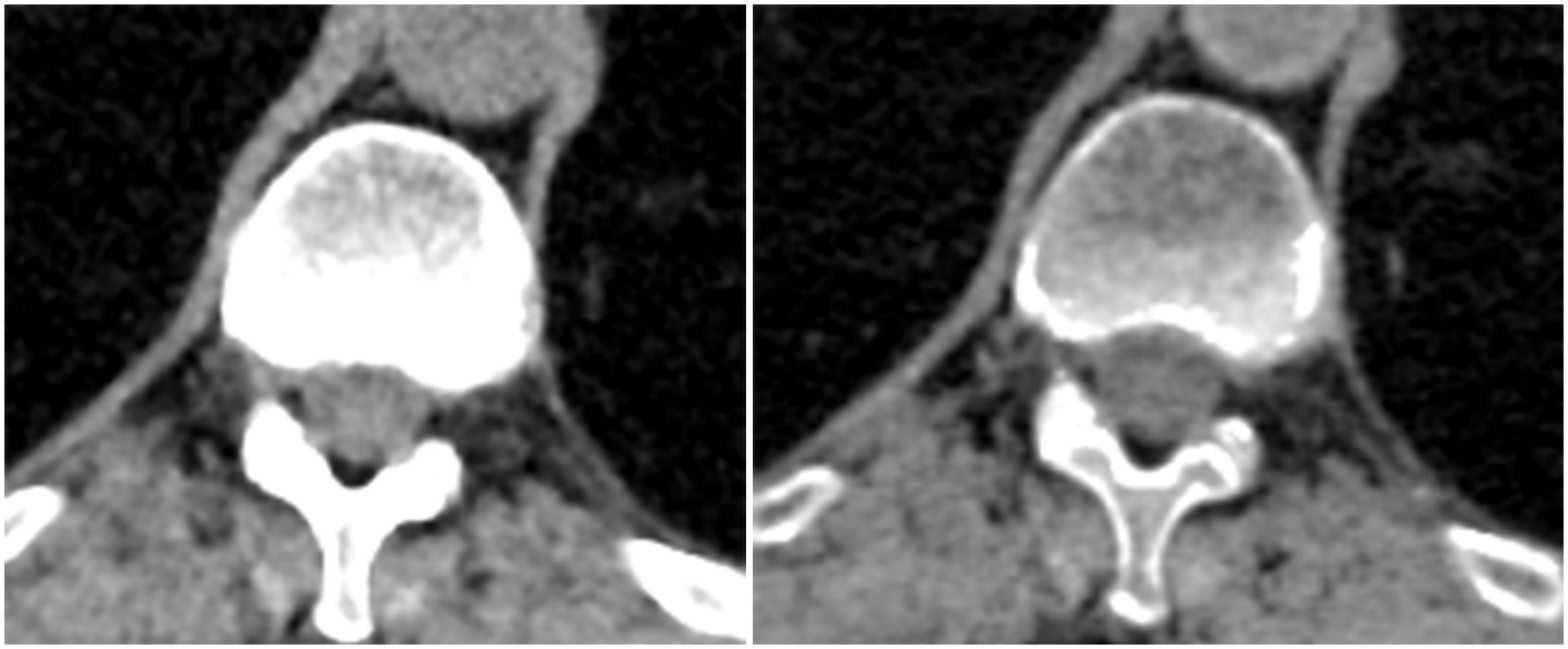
Example of calcium subtraction artifact. Same location in true unenhanced (TUE) image (left) and virtual unenhanced (VUE) image (right). Images are displayed using the same CT window width and window level.
